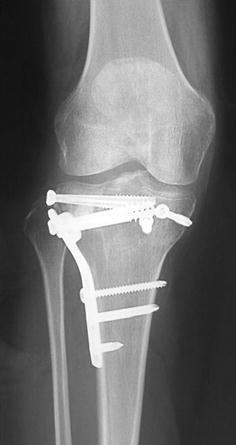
Schatzker type V fracture, 4 months X-rays (AP)
